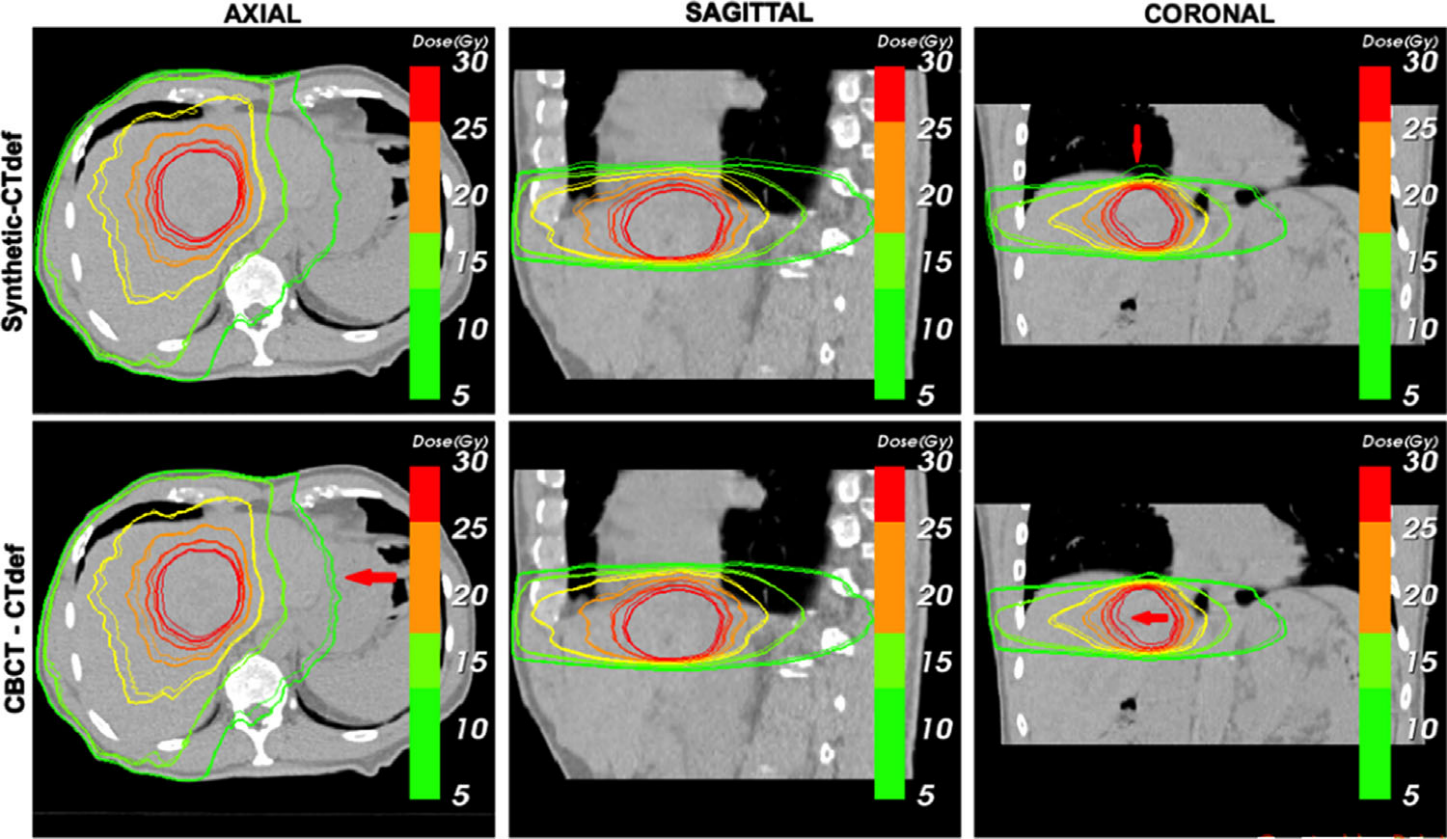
Isodose lines comparison superimposed to the deformed computed tomography (CTdef) image. The thick solid line corresponds to the reference computed tomography (CT)‐based dose calculation while the thin solid line corresponds to the synthetic‐based isodose (upper) and the cone beam computed tomography (CBCT)‐based isodose (lower). Red arrow points to regions of significant divergence (*d* > 2 mm).
